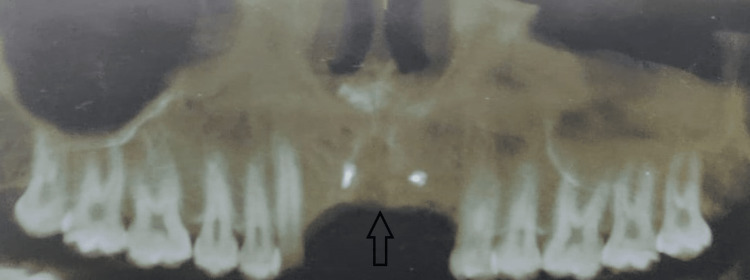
Postoperative CBCT after six months of the first surgical procedure CBCT: Cone-beam computed tomography
